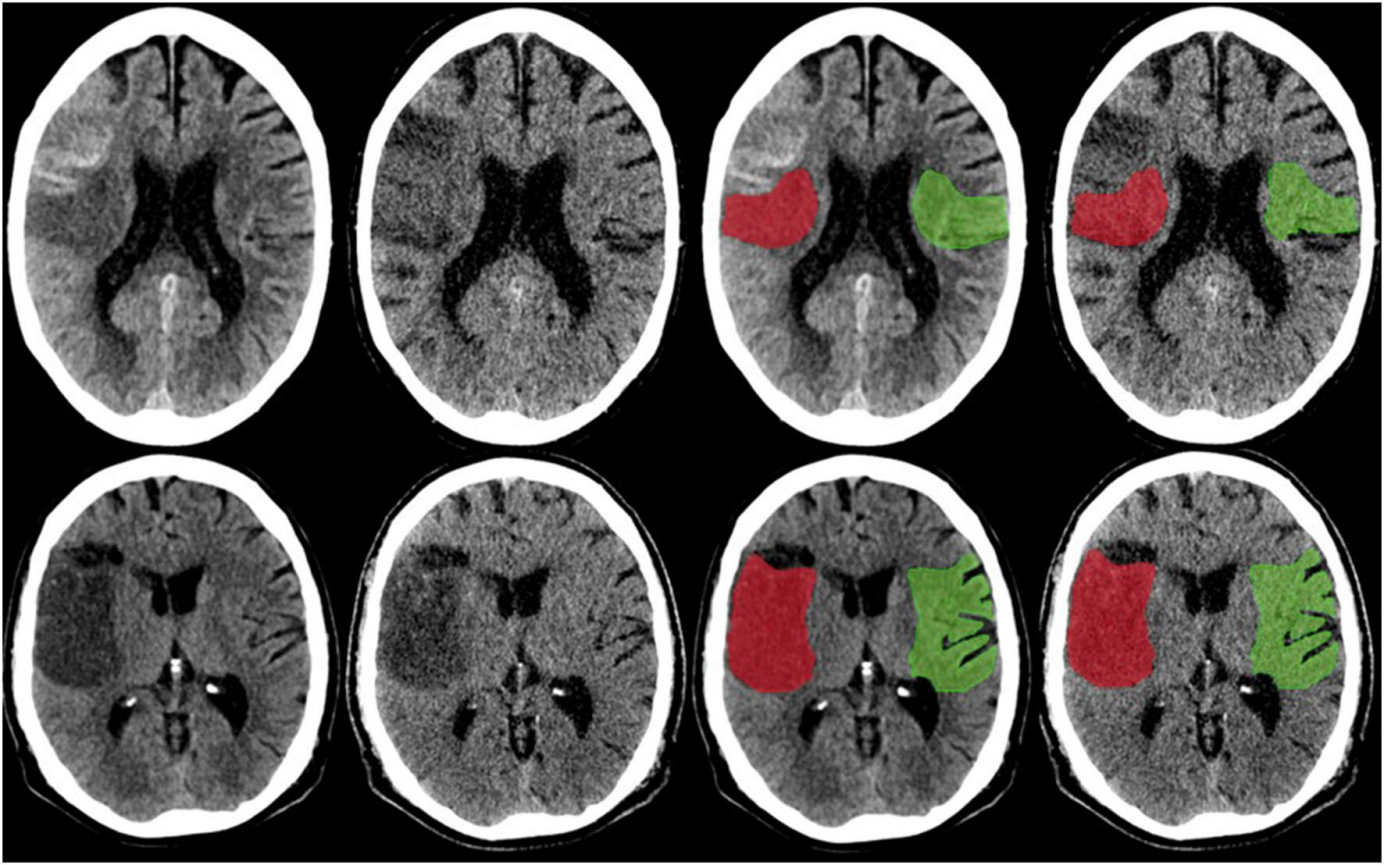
Examples for region of interest (ROI) placements in polychromatic (1st and 3rd images from left) as well as corresponding virtual non-contrast (2nd and 4th images from left) images for net water uptake measurements. The ischemic infarction (Red) was compared to the corresponding unaffected contralateral region (green). Top row: Follow-up dual-energy CT within 16 h in an 84-year-old female with right middle cerebral artery (MCA) occlusion (initial NHISS 20; ASPECTS 7) after thrombectomy (mTICI 0). Example to demonstrate how visually apparent hyperattenuations in conventional polychromatic images (CP-I) were spared during ROI placement. Edema underestimation was 12.8%. Bottom row: Follow-up dual-layer dual-energy CT within 16 h in a 69-year-old man with right MCA occlusion (initial NHISS 17; ASPECTS 4) after thrombectomy (mTICI 2b). Example of ischemic ROI placement if no visually apparent hyperattenuations were seen on CP-I. Edema underestimation was 4.5%.
